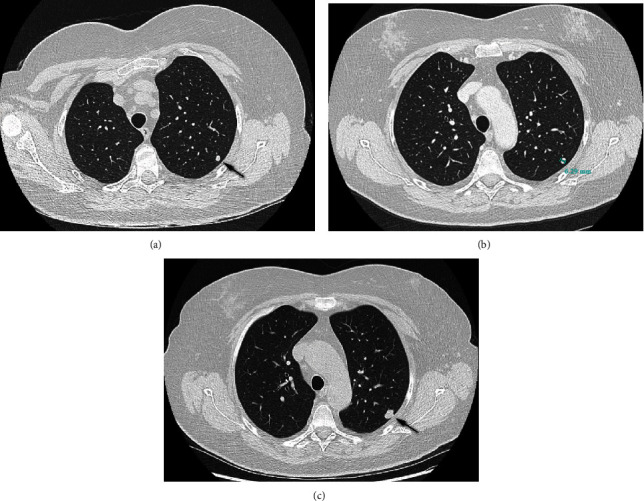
Radiographic images of the suspected metastases. CT scan of a dense opacity in the left upper lung lobe of 6 mm in April 2016 (a). The nodule was unchanged after 6 months of follow-up (b). In December 2019, the nodule increased to 13.5 mm (c).
